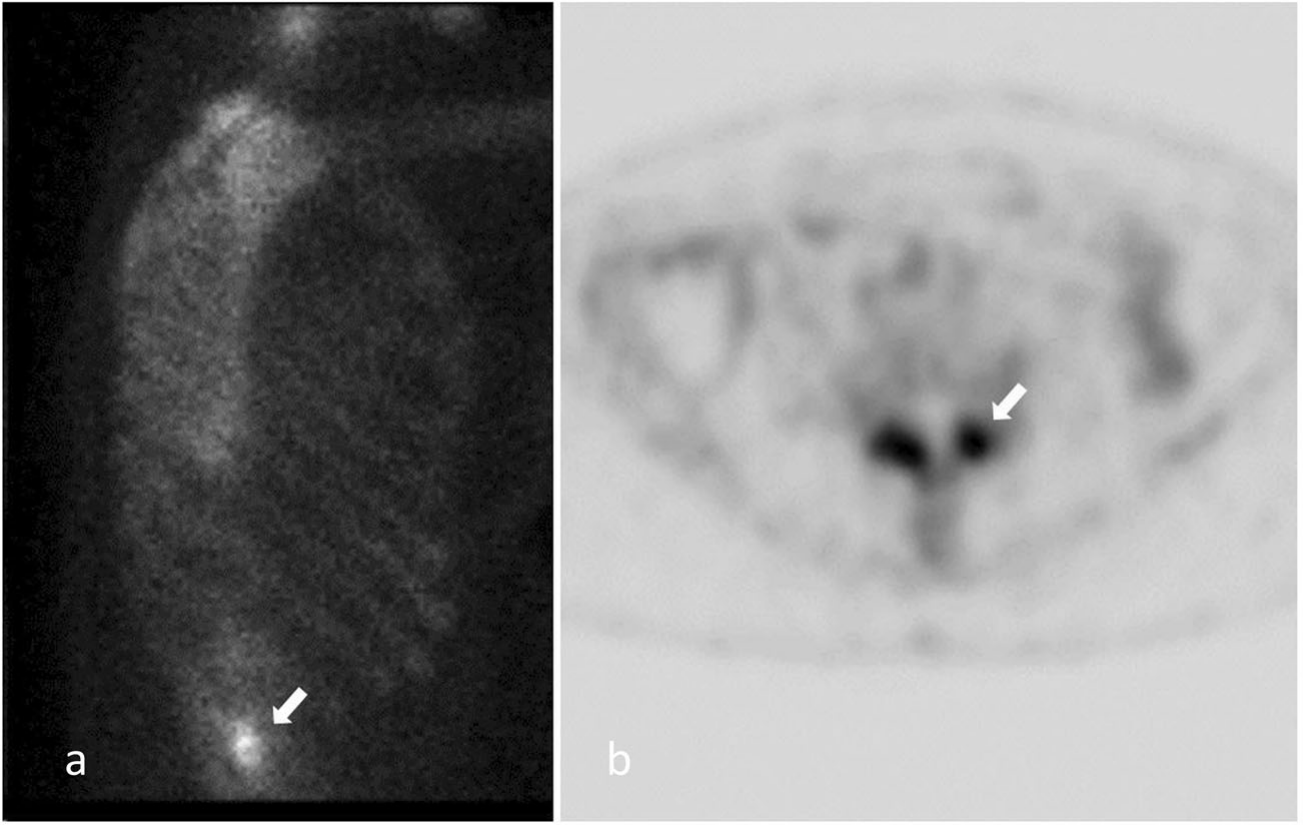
SPECT imaging of FJ. Hyperfixation on bone scintigraphy located on FJ capsule inflammation (white arrow)
